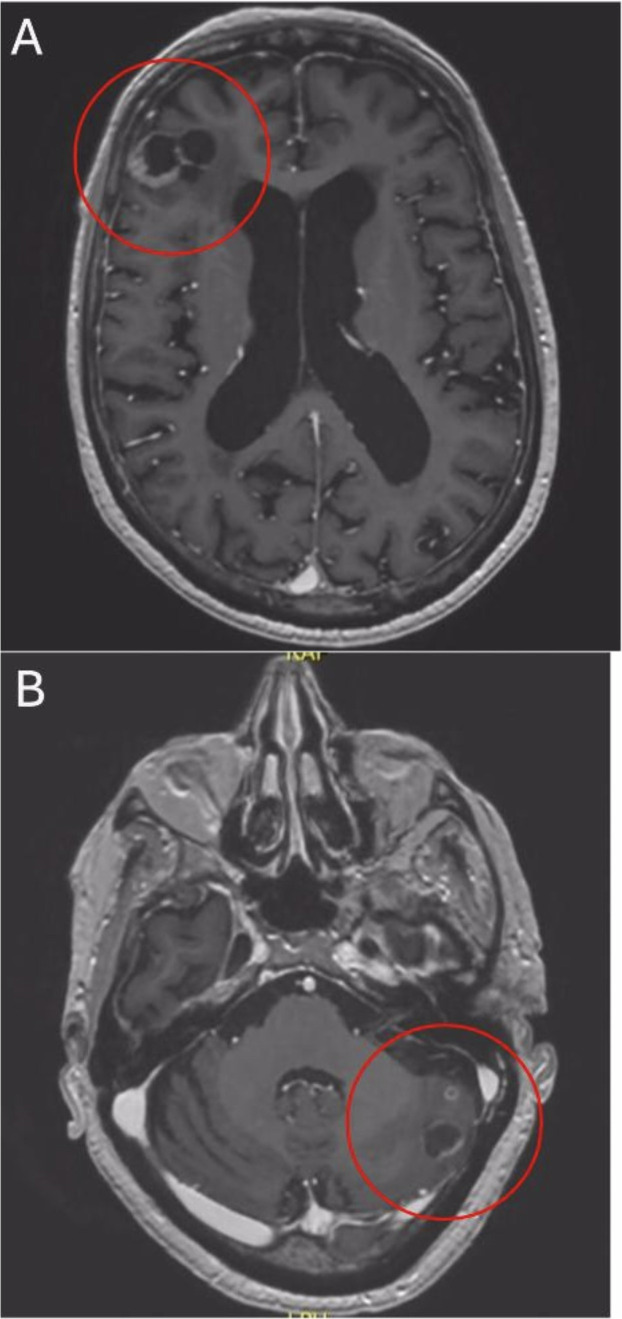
After 5 weeks of treatment axial T1-weighted MRI reveals remission of right frontal (A) and left cerebellar (B) lesions.
